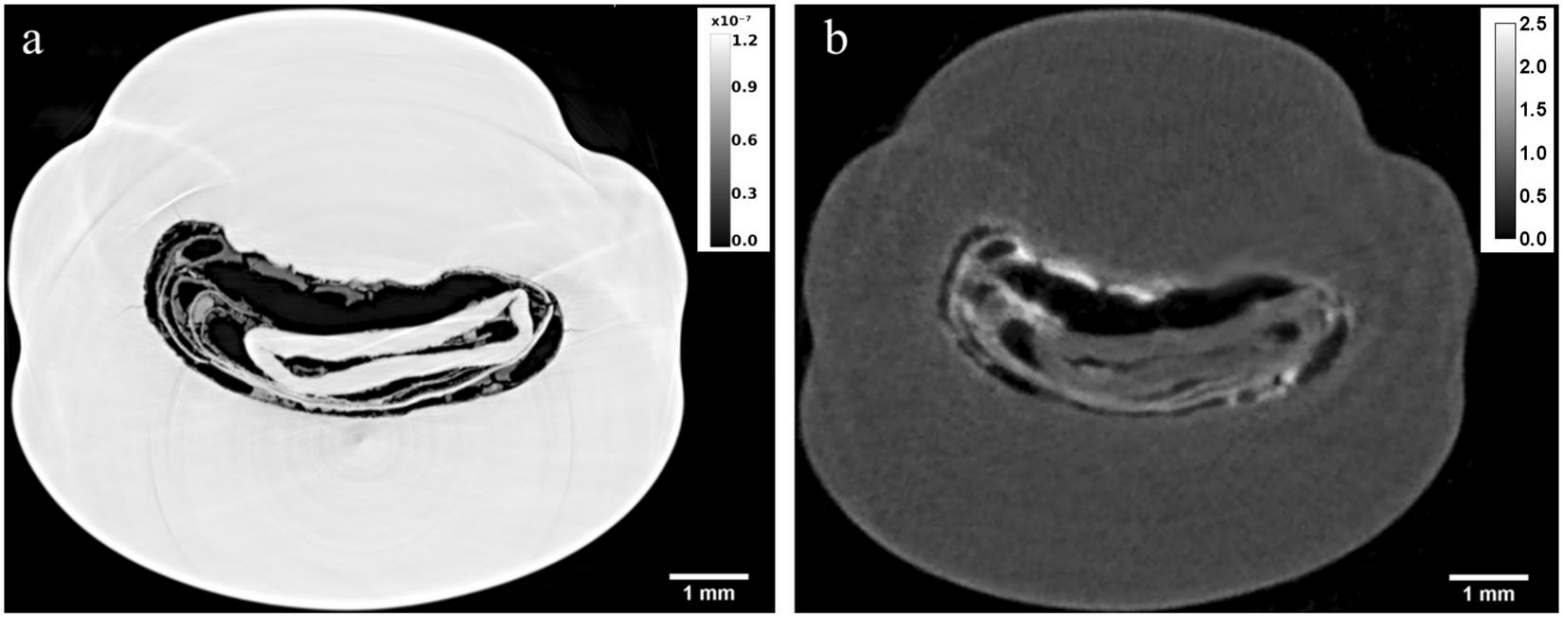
X-ray PC (a) and neutron (b) reconstructed image corresponding to the same slice of the natural pearl NP-22. Aragonite phases figure as white and dark grey zones in the X-ray and neutron images, respectively. Organic matter is recognized as dark grey and white zones in the X-ray and neutron images, respectively. In the neutron image an elevate concentration of organic matter has been identified, visible as white zones due to hydrogen presence. On the other hand, the X-Ray PC image shows with more details the concentric growth arcs and the layers of organic matter. Grey scale refers to refractive index decrement for PCI (a) and to linear attenuation coefficient (cm^−1^) for NI (b).
